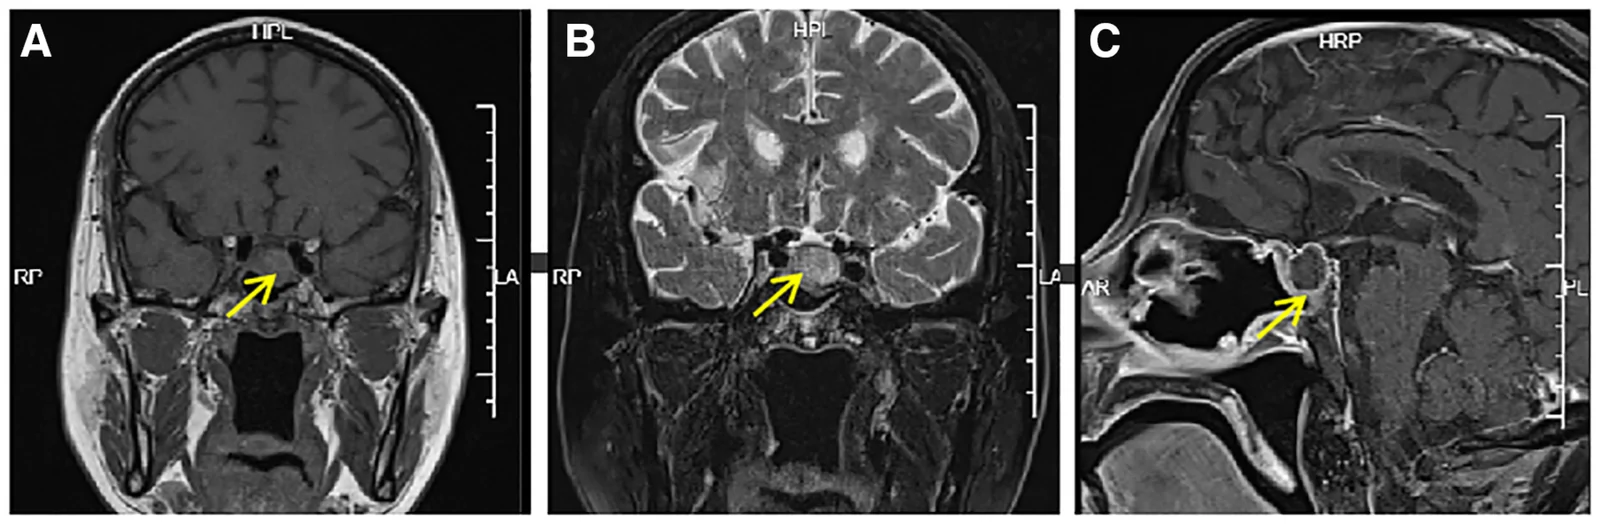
Postoperative pituitary-enhanced MRI. (A) Coronal T1-weighted images, (B) Coronal T2-weighted images showed mixed abnormal signals on the left side of the sella turcica, which broken through the sella turcica septum upward. (C) Sagittal T1-weighted images showed rim enhancement after gadolinium injection (highlighted by the arrow).
